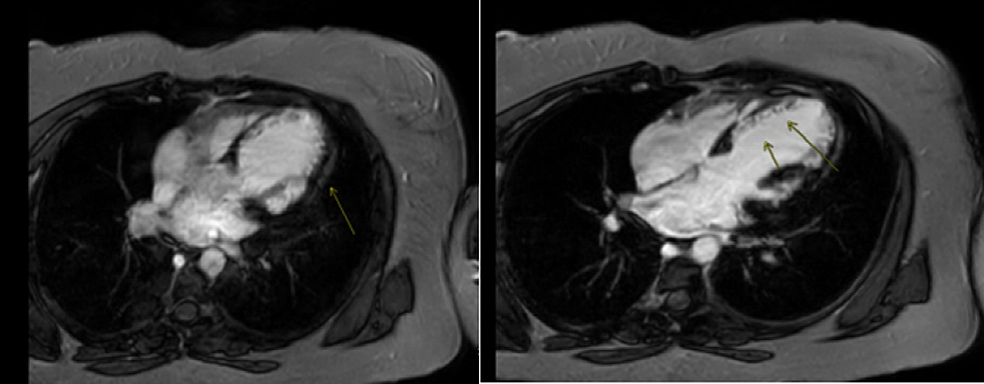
Cardiac MRI Endocardial enhancement along the apical anteroseptal wall with edema in keeping with acute infarction, this is greater than 50% thickness of the septum
